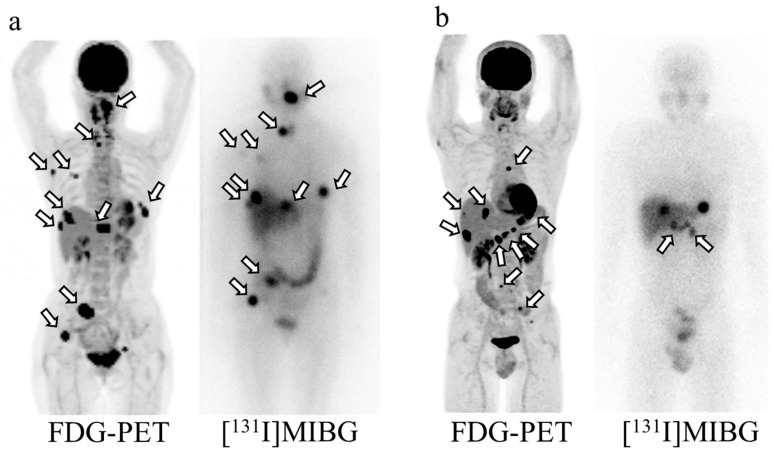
Representative cases. (a) The MIP image of the FDG-PET prior to [^131^I]MIBG treatment of a 65-year-old woman with bone and lymph node metastases. The urine catecholamines included adrenaline (14.3 μg/day), noradrenaline (1110.2 μg/day), and dopamine (989.2 μg/day). All of the metastases showed apparent uptake on scintigraphy after [^131^I]MIBG treatment. (b) The MIP image of the FDG-PET prior to [^131^I]MIBG treatment of a 45-year-old man with lymph node, liver, and bone metastases. The urine catecholamines included adrenaline (7.9 μg/day), noradrenaline (647.5 μg/day), and dopamine (2115.9 μg/day). Only a few lymph node metastases showed apparent uptake on scintigraphy after [^131^I]MIBG treatment_._ MIP, maximum intensity projection; FDG-PET, fluorodeoxyglucose-positron emission tomography; MIBG, metaiodobenzylguanidine.
